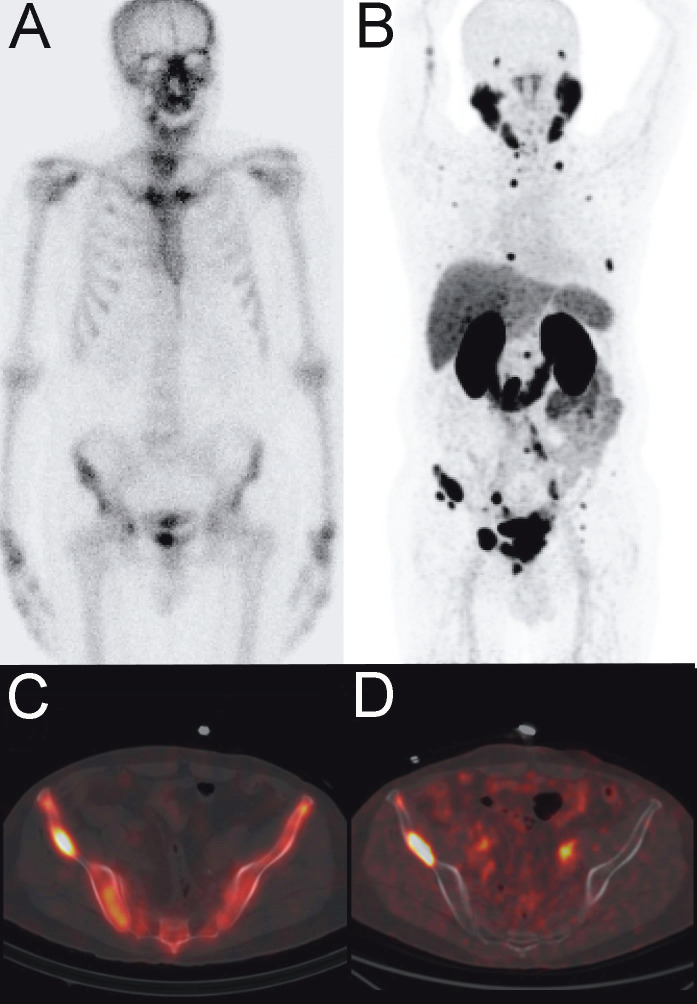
Tc-99m MDP bone scintigraphy planar (A), and fused SPECT/CT (C) images
